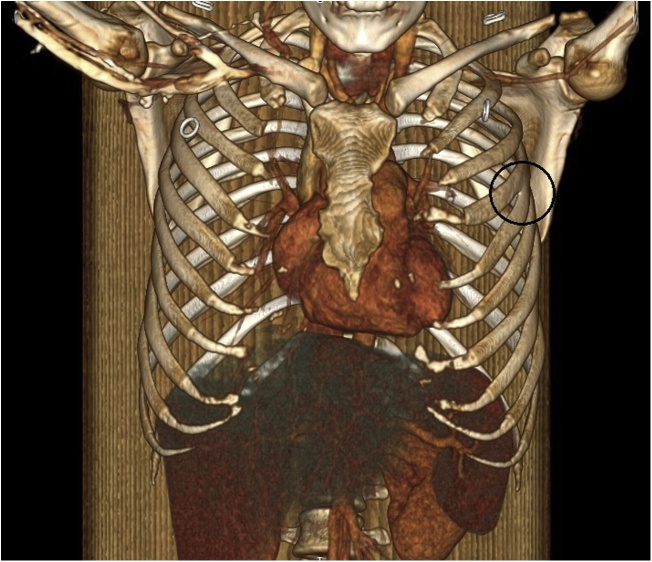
Three-dimensional volume rendering computed tomography (3D-CT) image demonstrates a left 4th bifid rib (encircled).
